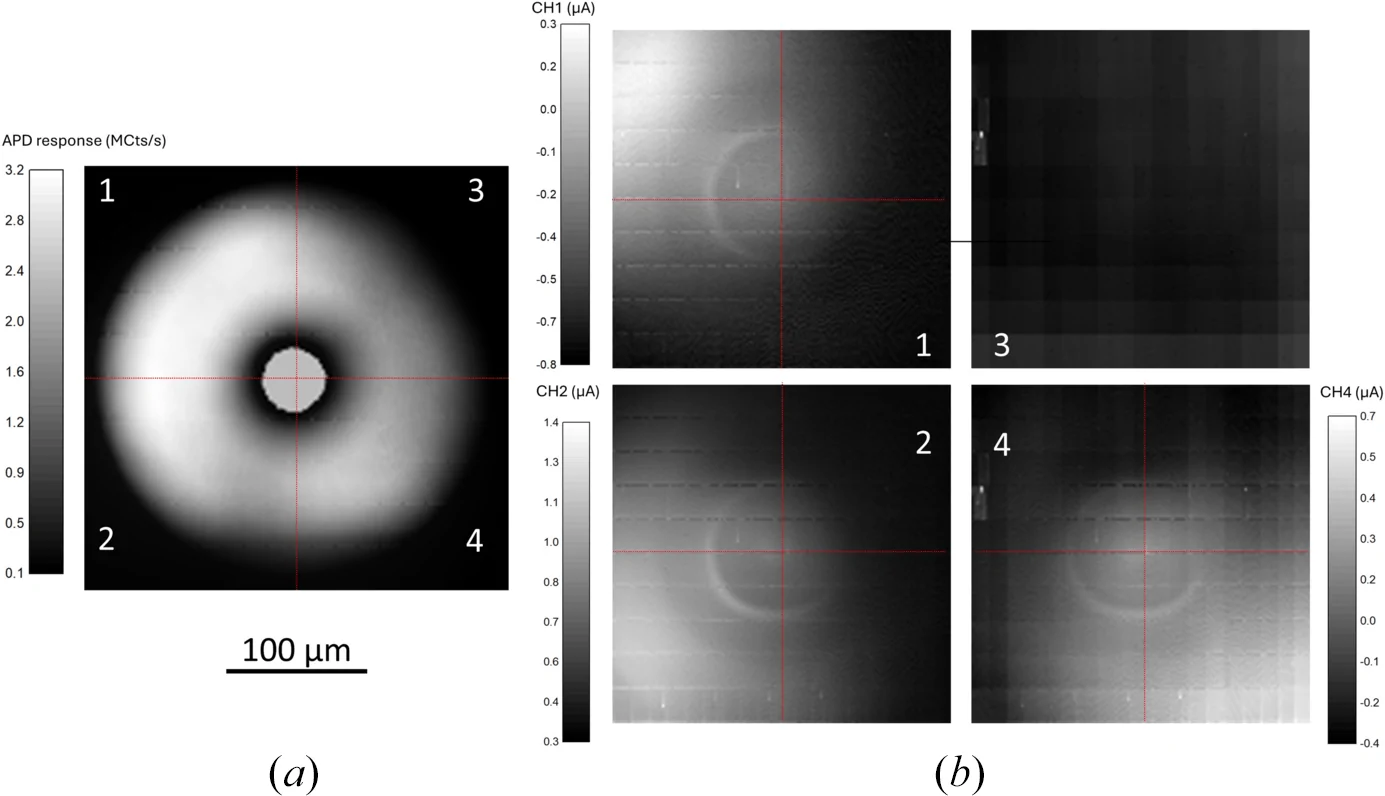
(*a*) STXM image of the X-ray beam illuminating the SiC OSA, where the zeroth-order focus (outer ring) and the first-order focus (central disk) can be observed. (*b*) Response of the four individual sectors of the SiC diode [marked in (*a*)] achieved by scanning the OSA across the beam defined by the Fresnel zone plate installed in the PolLux STXM. The response of each channel, as expected, is larger when the beam irradiates the corresponding diode sector. Sector 3 shows no response due to a faulty contact.
